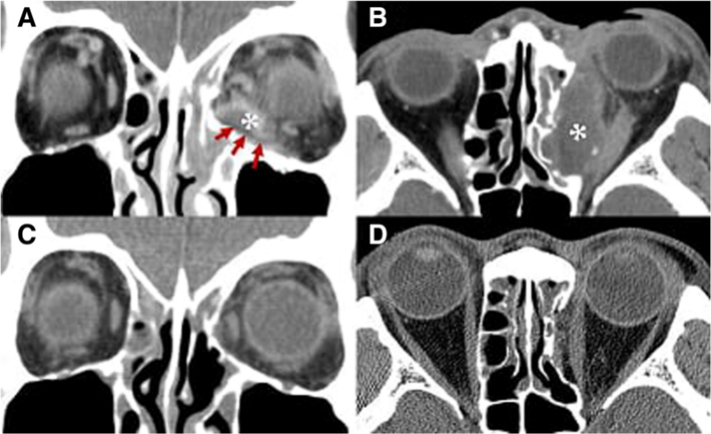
Patient 3. a Coronal CT showed a D-shaped low density mass (asterisk) adjacent to the radiolucent sheet (arrows). Sinus opacification was evident in the frontal and ethmoid sinuses. b An axial CT image showing a large low density mass (asterisk) extending to the entire medial wall of the orbit. c, d Five months after the explantation and sinus surgery
